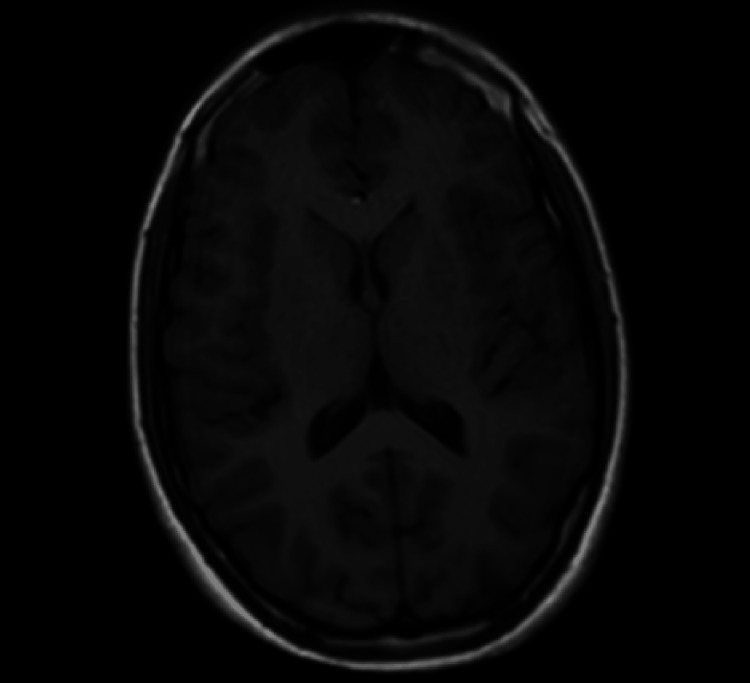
Normal brain MRI MRI: Magnetic resonance imaging
